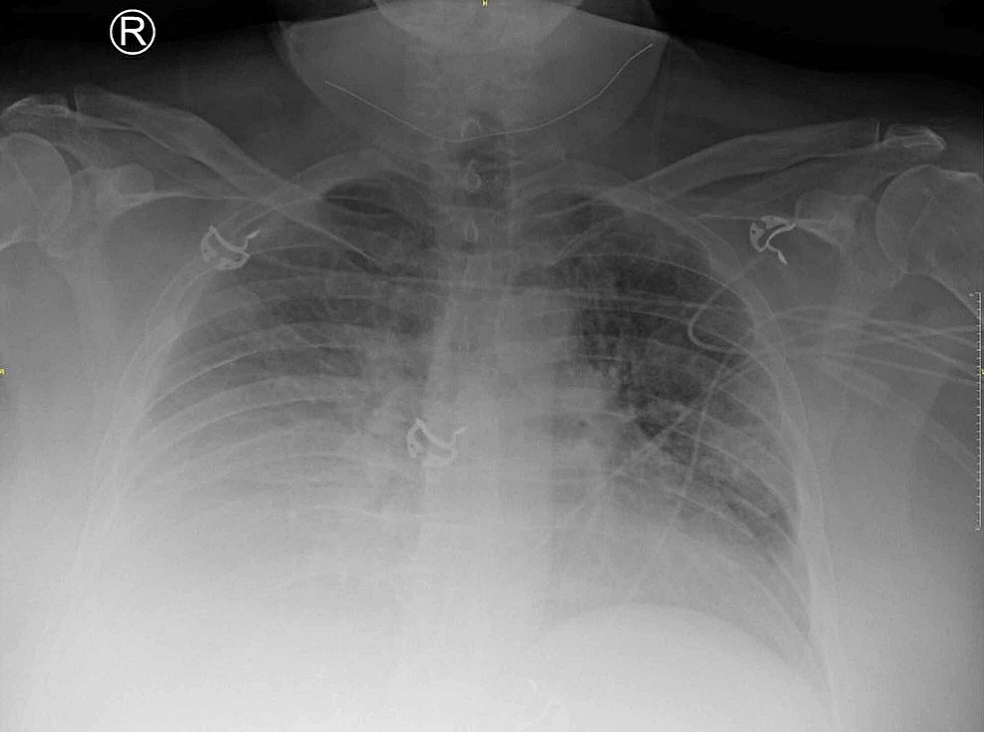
Chest X-ray on presentation Anterior-posterior (AP) view showing opacification of the right hemithorax consistent with right pleural effusion and right basilar atelectasis.
